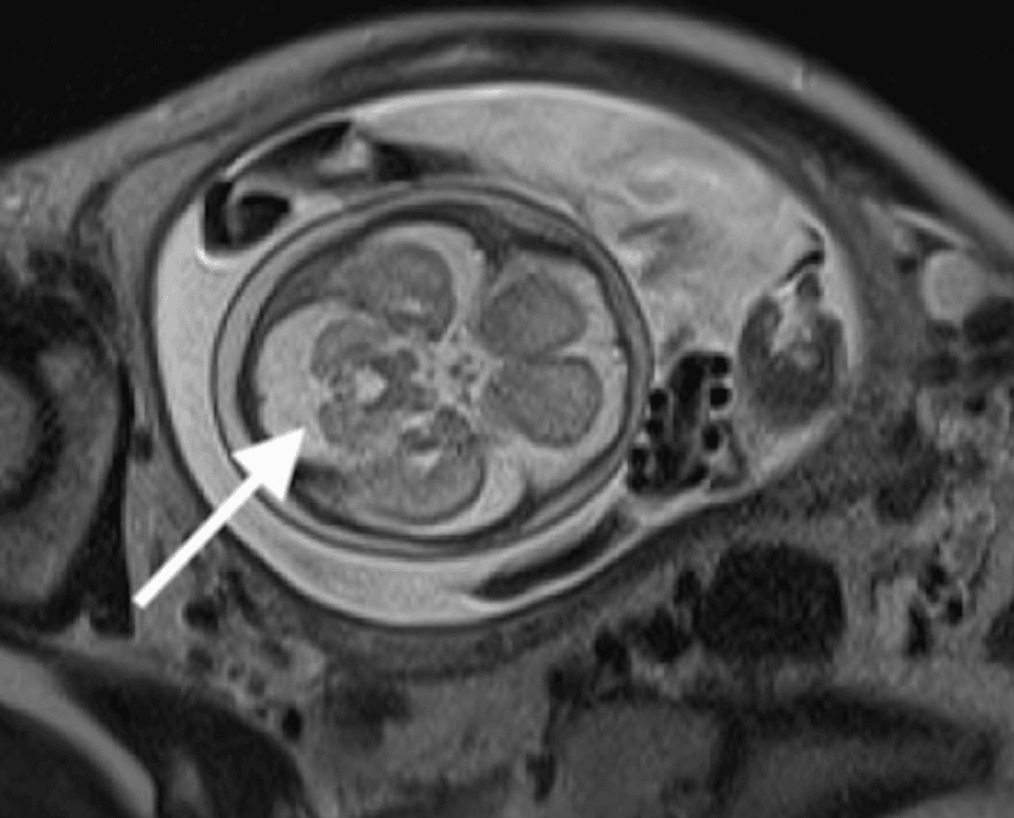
Fetal magnetic resonance imaging findings (T2-weighted image). The transverse image of the brain of the fetus shows the molar tooth sign (thickening of the upper cerebellar peduncle: white arrow).
